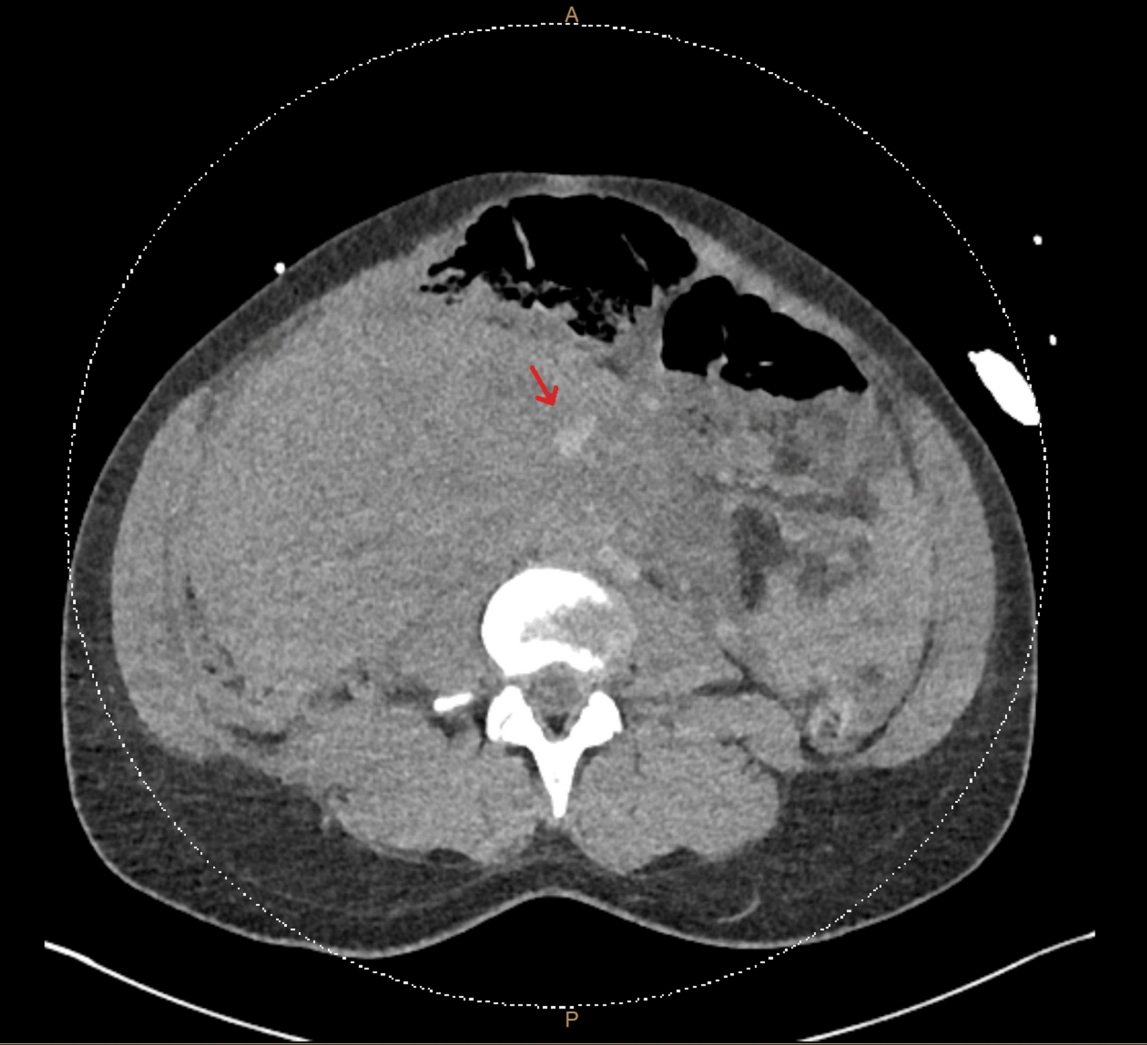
Transverse view of the repeat CT angiogram on arrival to the tertiary hospital, with the red arrow showing contrast extravasation on delayed-phase imaging.
